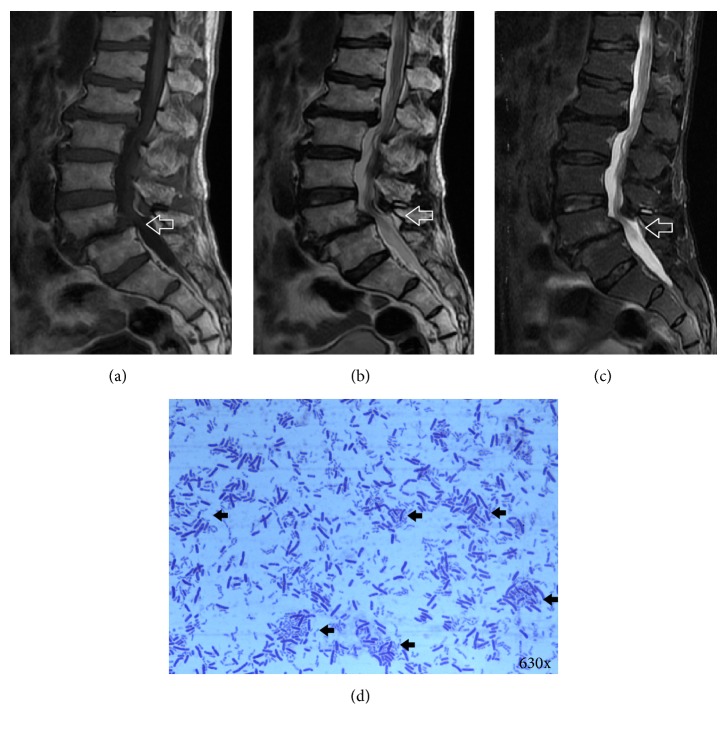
P. acnes* identified and isolated from a nonpyogenic intervertebral disc. (a)–(c) A 68-year male patient (patient's ID: number 9) underwent discectomy due to severe low back pain and sciatica. The disc harvested from the patient at level of L4~5 was proved to be* P. acnes*-positive using anaerobic culture and 16S rDNA PCR. The signs of discitis, such as osteolytic changes of endplates or vertebrae, pyogenic changes of disc, and septic changes of vertebral surrounding soft tissues, were not seen in T1WI (a), T2WI (b), and STIR (c) of MRI (marked with white blank arrows). (d) Rod-shaped, Gram-positive bacterium was isolated and purified from the* P. acnes*-positive disc (marked with black arrows) and the purified bacterium was* P. acnes*.
